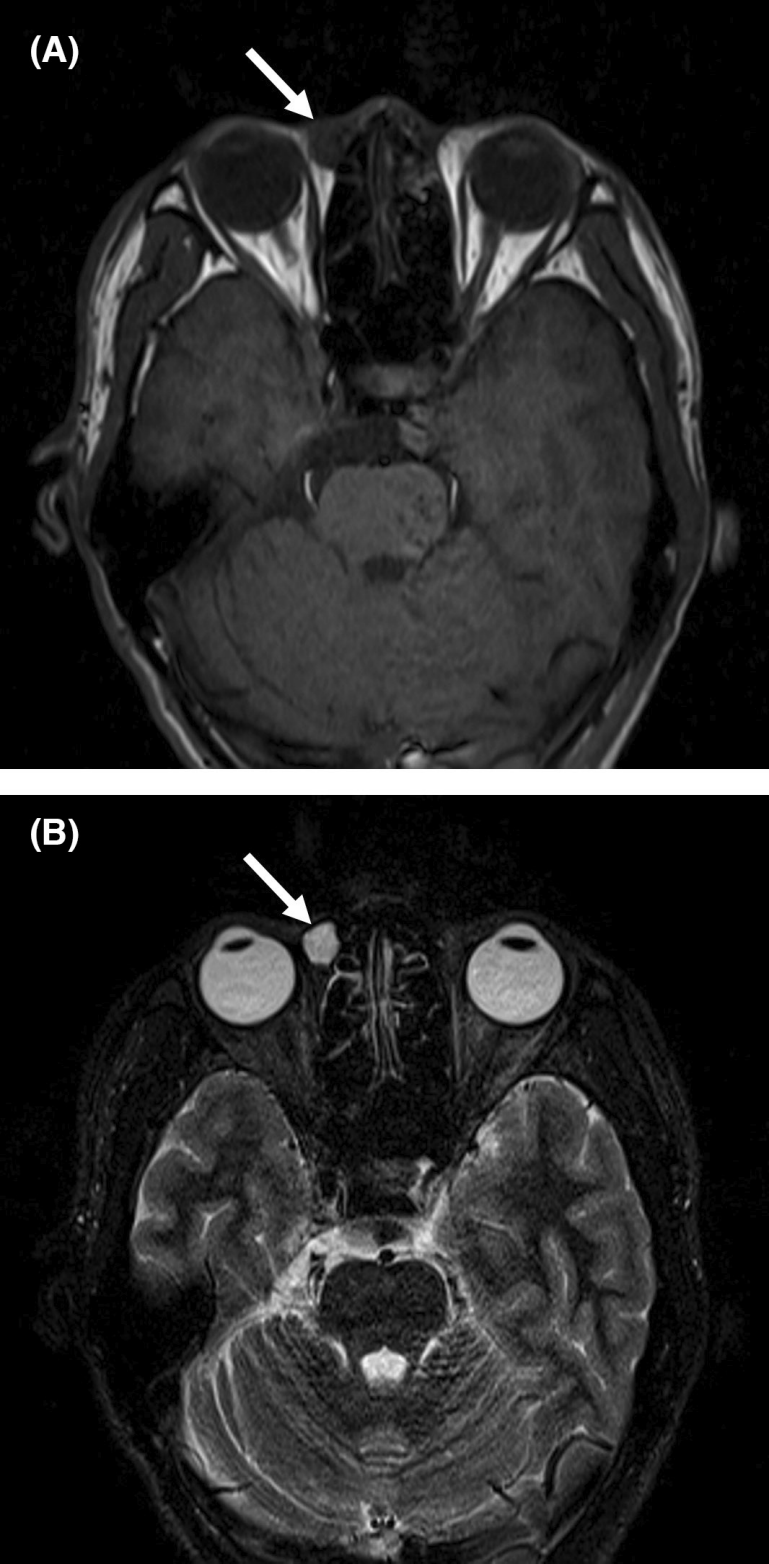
Magnetic resonance imaging of superficial angiomyxoma. (A) The tumor (arrow) near the right lacrimal sac was circular and defined on T1‐weighted images. (B) T2‐weighted images showed that the tumor (arrow) had long signals.
